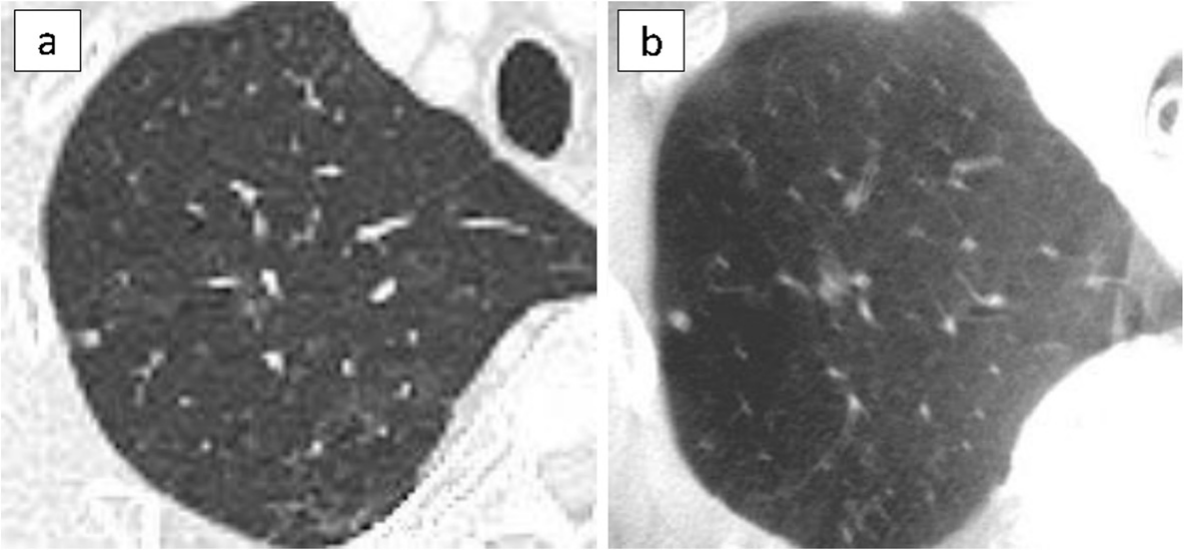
A small nodule. Preoperative CT shows a small nodule, 3 mm in diameter (a). The O-arm shows an obvious nodule (b).
